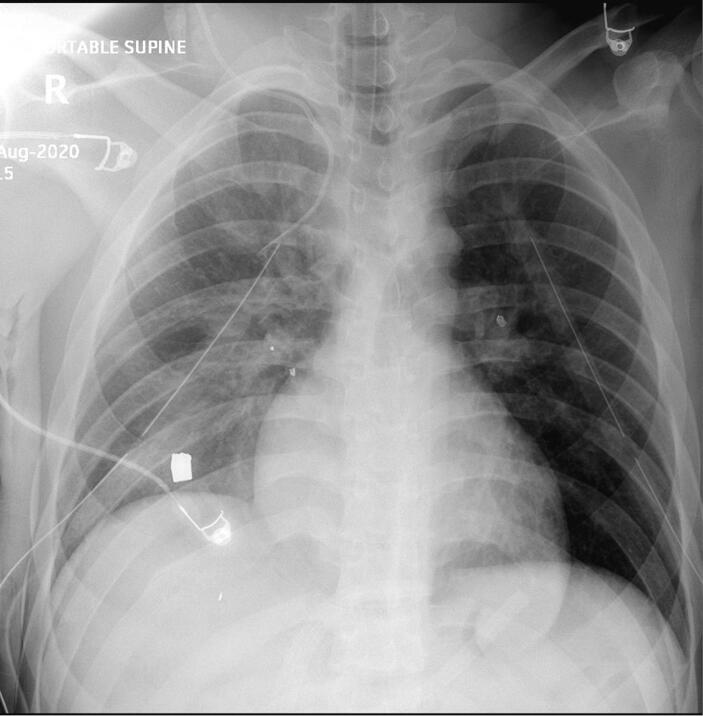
Chest X-Ray reveals malpositioning of the central venous catheter on the right.
